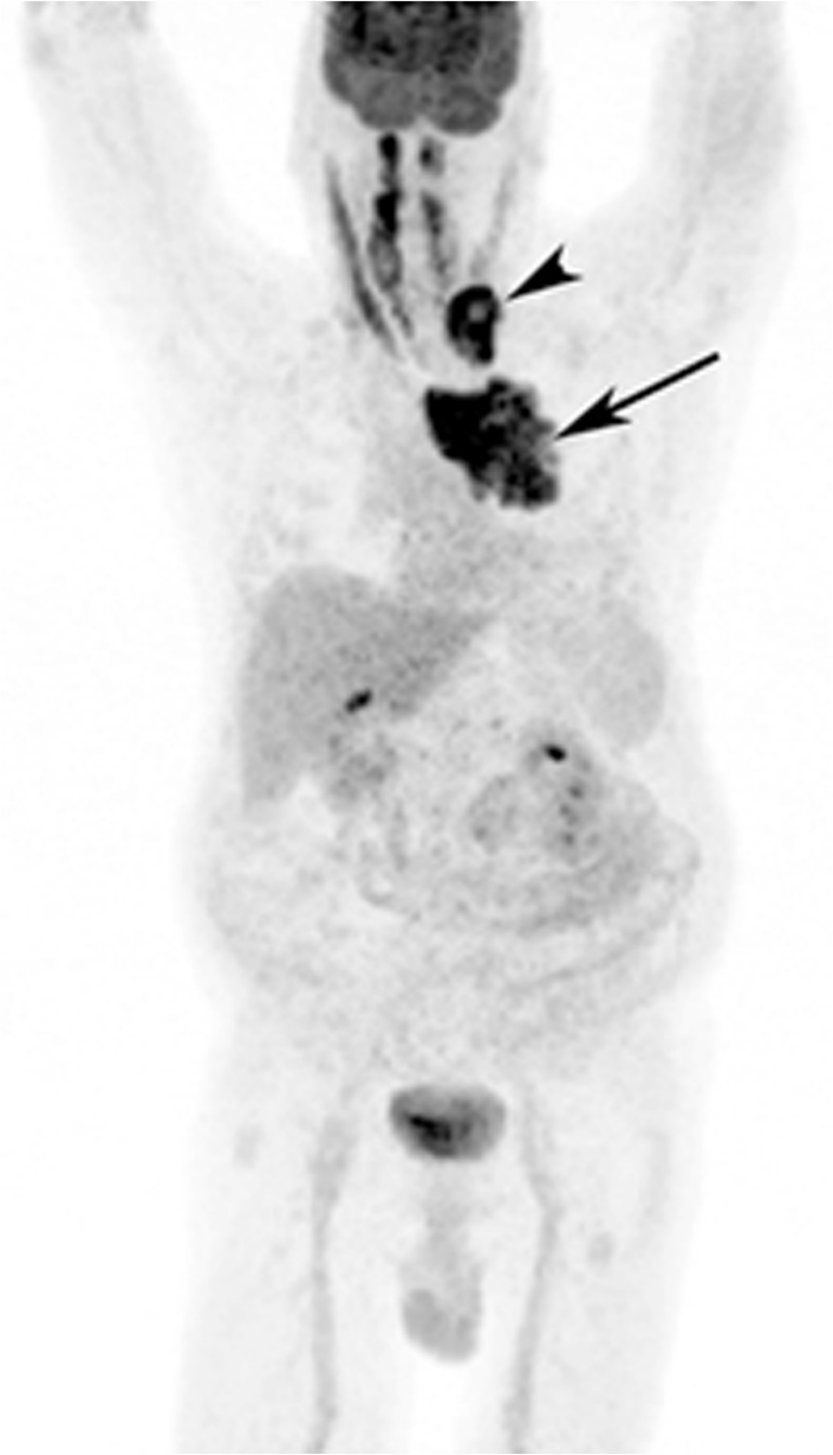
Whole body PET scan using 18 F-FDG. PET scan shows a left upper thorax tumor mass (arrow) and metastases to left supraclavicular lymph nodes (arrow head).
